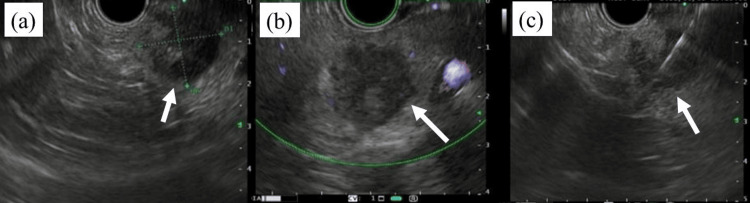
Endoscopic ultrasonography (EUS) (a) EUS image showing a 20-mm-in-diameter circular hypoechoic mass in the pancreatic tail (arrow). (b) A hypoechoic lesion is observed (arrow). (c) EUS-guided fine-needle aspiration revealing a poorly differentiated adenocarcinoma that differentiated into the squamous epithelium (arrow).
